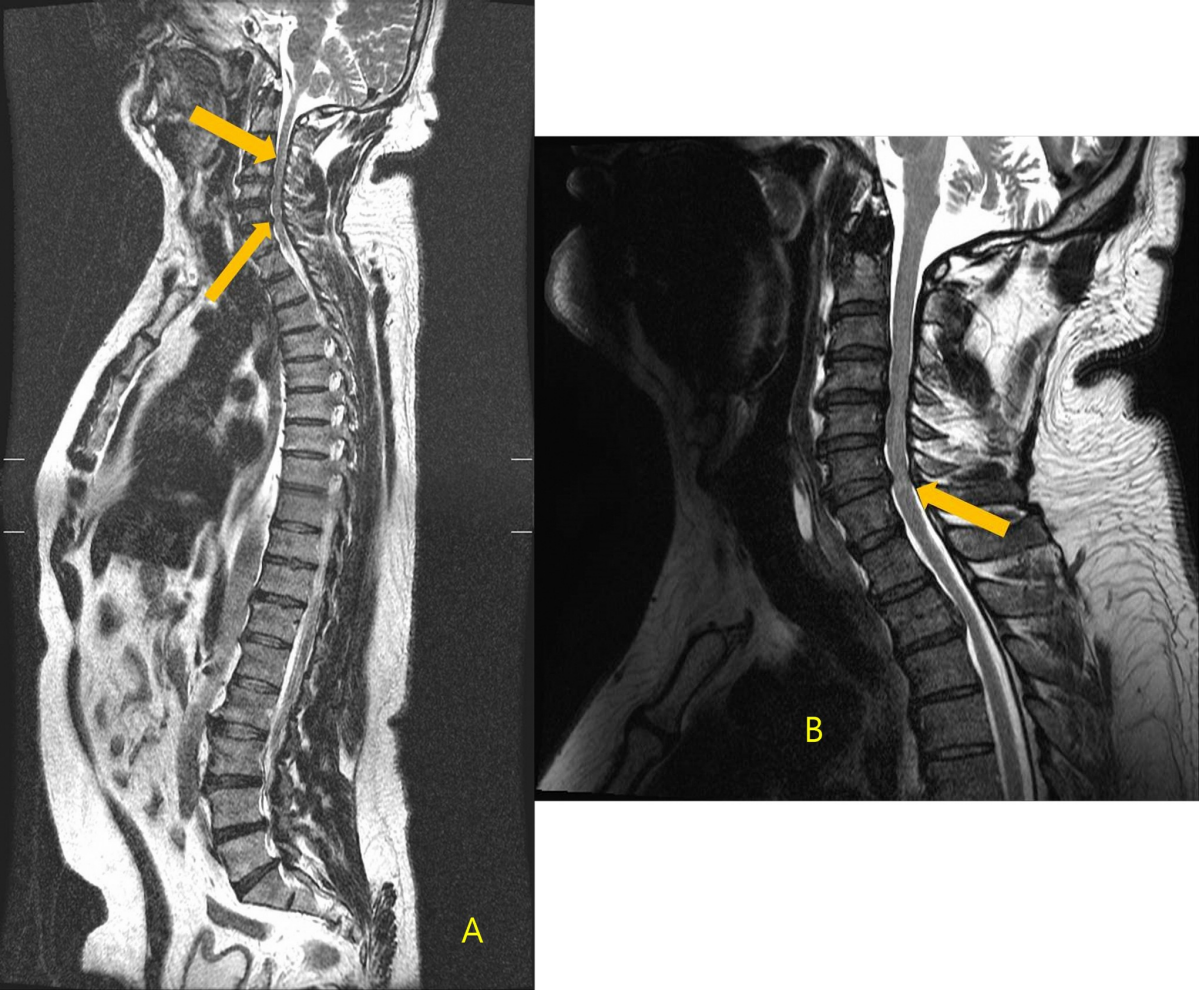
A 44-year-old man who underwent a routine health examination. A: Whole-body sagittal T_2_ fast spin echo (TR/TE, 4000/110) revealed a disc protrusion at C3-4 and C6-7 (arrows). A faint bright signal was seen in the spinal cord (thin arrow). The initial radiologic diagnosis was severe disc protrusion. Radiologist reviewers classified this lesion as category II.B: On the follow-up sagittal T_2_-weighted MR image (TR/TE, 300120) of the C-spine, definite myelopathy was seen in the spinal cord at the C6 level (arrow).
